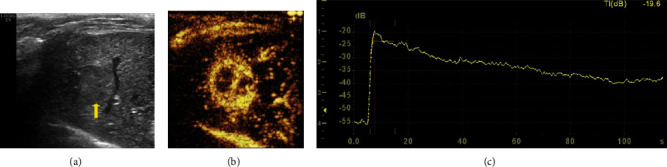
Ultrasound image of VX2 liver tumor in rabbits. A gray-scale ultrasound (a) showed a single sphere-alike homogeneous hypoechoic nodular with a clear boundary (arrow). The CEUS (b) and time-intensity curve (c) of VX2 liver tumor showed a characteristic “rapid wash-in and wash-out” vascular perfusion pattern.
